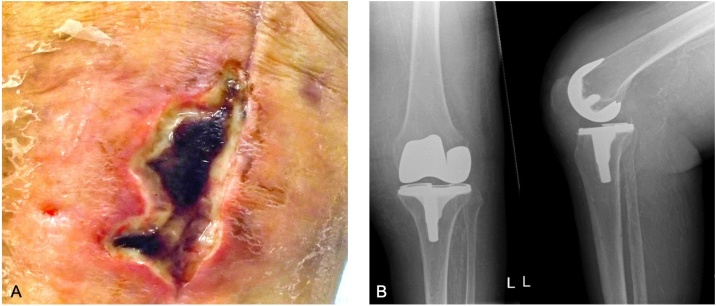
Knee of the patient with wound healing disorder (A) and x-ray images after primary total knee endoprosthesis implantation (B).
